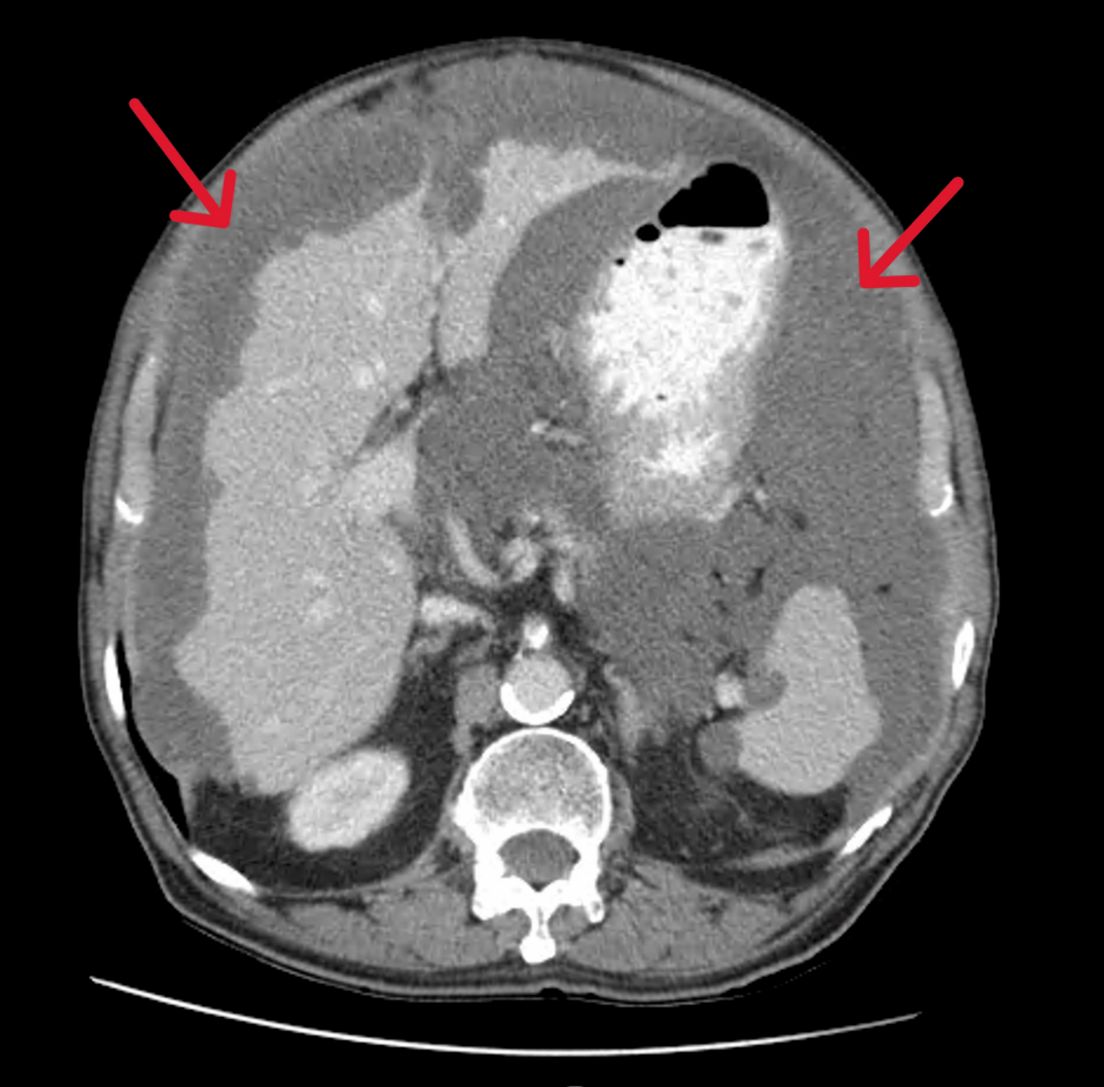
Abdominal CT-Scan (axial view) The red arrows point to the pseudomyxoma's involvement.
